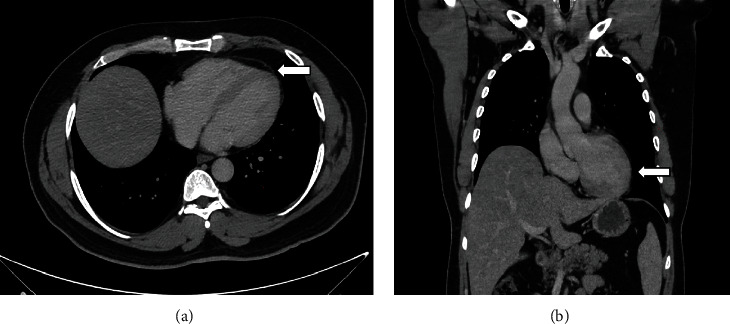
Enhanced CT scan of the chest after a complete course of colchicine and ibuprofen showing improvement of the pericarditis evident by significant improvement of the pericardial thickening and resolution of the pericardial and left pleural effusions.
